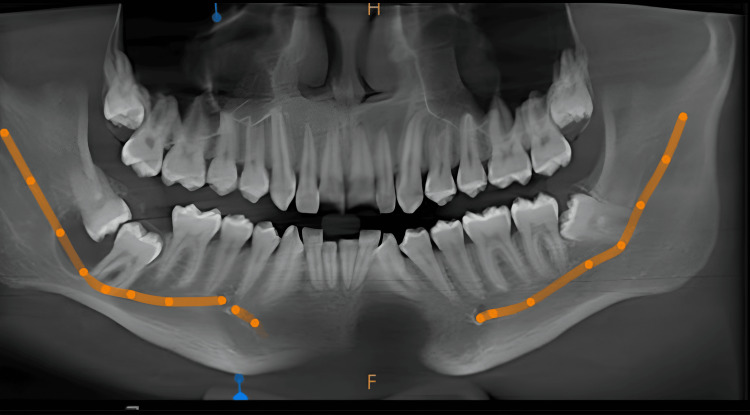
CBCT showing a well-defined unilocular radiolucency surrounding the crown of horizontally impacted 48 CBCT: Cone beam computed tomography
